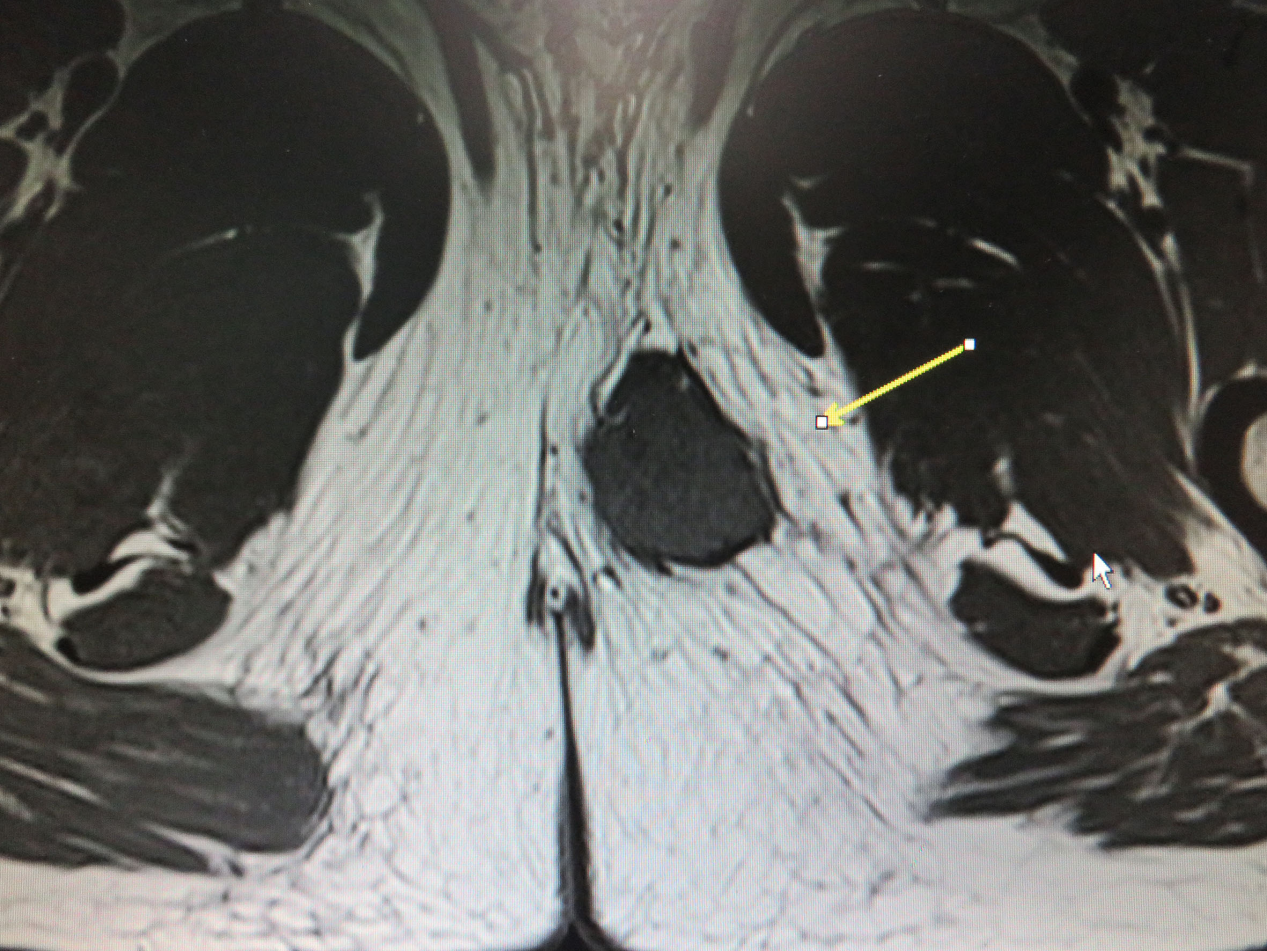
MRI revealed a perineal tumor. The arrow points out the location of the lump.
